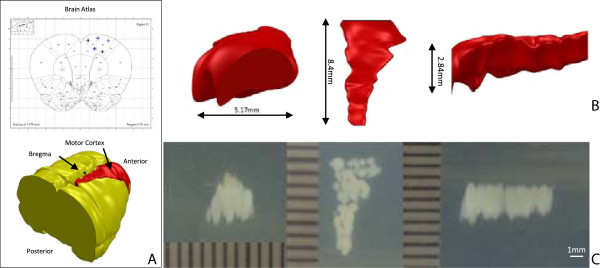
Results obtained for generating a 3D ultrasound exposure pattern. (A) A
